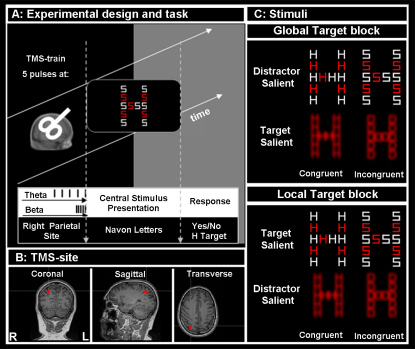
Experimental Design, Task, Stimuli, and Stimulation Site (A) Experimental design and task. Rhythmic TMS was applied in short bursts of five pulses at one of two frequencies (theta band, 5 Hz; beta band, 20 Hz) on each trial in the main experiment, in random order across trials, with 10 s intervening between successive bursts. Onset of a global/local hierarchical visual stimulus, centered at fixation, coincided with the last TMS pulse of each burst so that the critical visual display closely followed the rhythmic TMS bursts (future studies might vary this timing to examine the temporal profile of our effects). A sham TMS condition was also conducted (coil tilted at 90° over the same parietal site), in separate blocks that were randomly intermingled with active-TMS blocks. (B) Stimulation site for one representative participant. TMS was applied over a right-hemisphere intraparietal sulcus site in our main experiment, determined by neuronavigation with Brainsight and individual anatomical MRI scans, at Tailarach coordinates 28, −51, 50 (see Results and Discussion and Experimental Procedures). (C) Examples of stimuli for the global/local target blocks. In the global target blocks, observers were asked to detect the presence (versus absence) of the global letter H (versus S or D). The local distractors were all Hs, or all Ss or Ds, independent of global identity, leading to equiprobable congruent and incongruent conditions. For blurred stimuli, the global letter was more salient than the local letters; the reverse was true for nonblurred stimuli. In other blocks of trials, the same stimuli were used, but the local level was judged instead. For both tasks, salient incongruent distractors interfered the most (see Supplemental Experimental Procedures and Figure S1).
